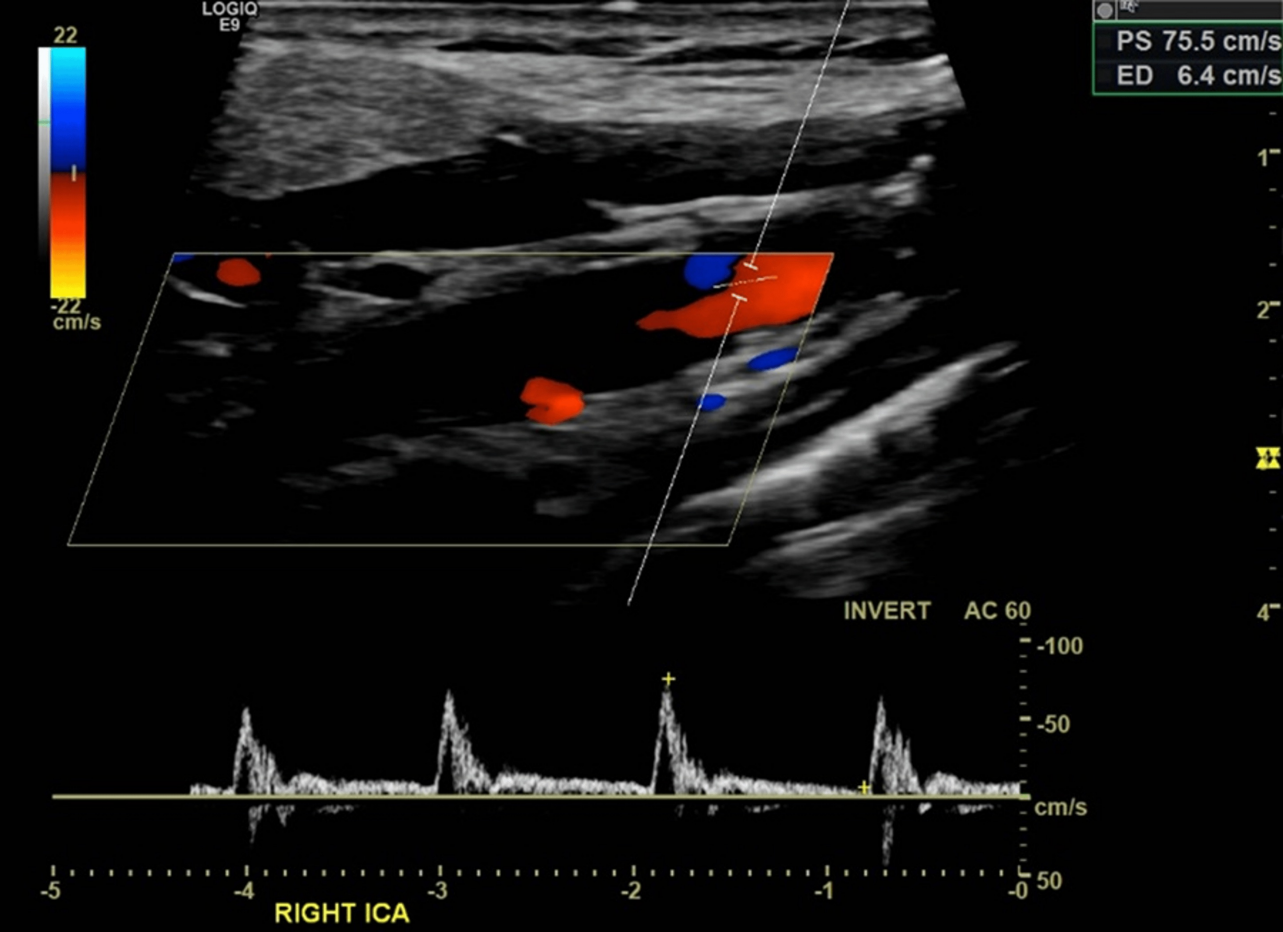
Doppler ultrasound of the right ICA demonstrated a lack of colour flow within the vessel, with a proximal 'flame sign,' along with bi-directional flow (red and blue signal within the vessel). ICA, Internal Carotid Artery
